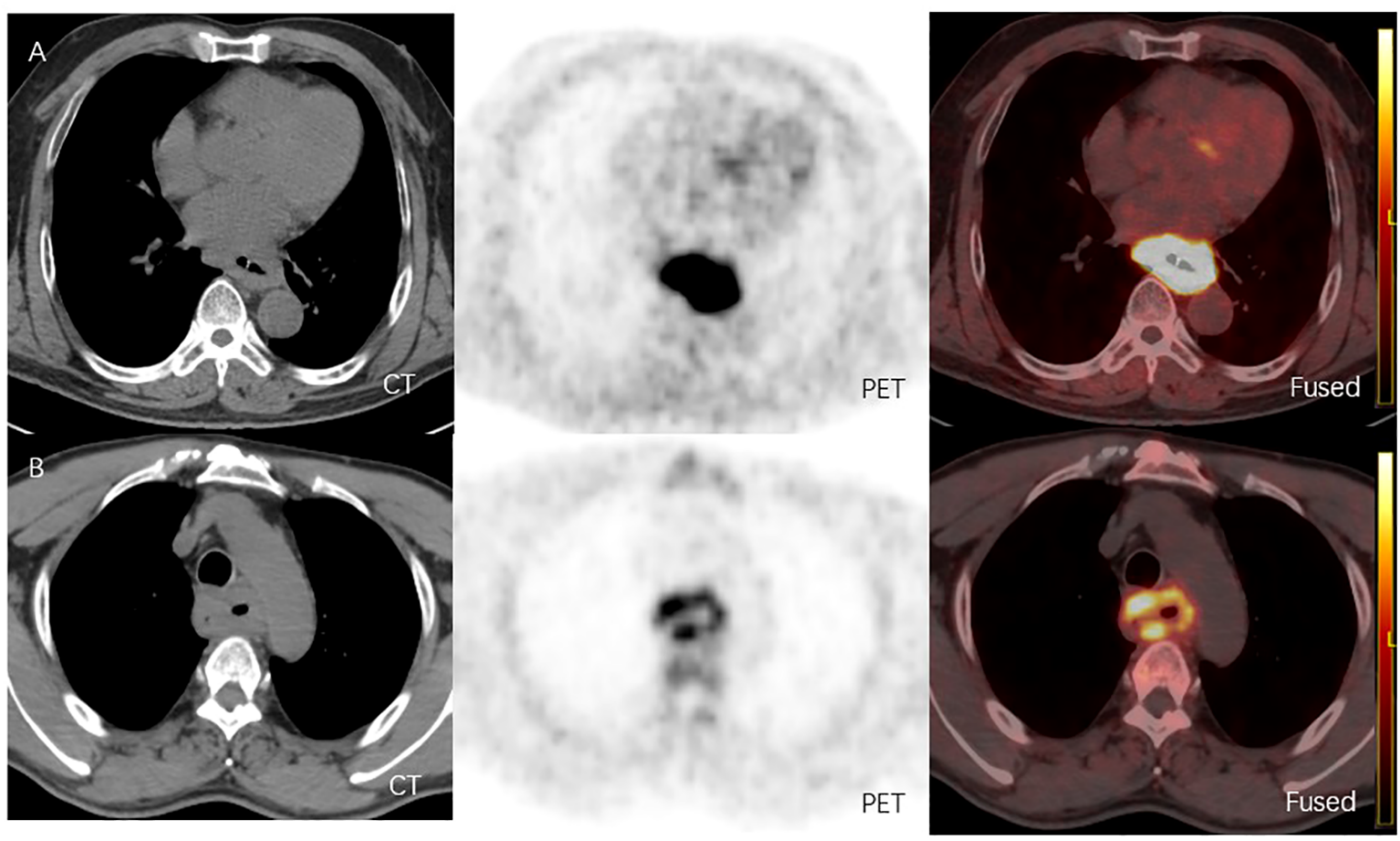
(A) 18F-FAPI-04 PET/CT and CT images of a LA-ESCC patient with an outcome classified as PD-L1 positive expression(CPS about 70), with SUVmax 15.36, SUVmean 8.60, SUVpeak 11.02, SUVsd 2.47, MTV 51.52 cm3 and TLF 443.07g. (B) 18F-FAPI-04 PET/CT and CT images of a LA-ESCC patient with an outcome classified as PD-L1 positive expression(CPS<1) , with SUVmax 6.45, SUVmean 3.36, SUVpeak 4.51, SUVsd 0.89, MTV 53.99 cm3 and TLF 181.41g.
